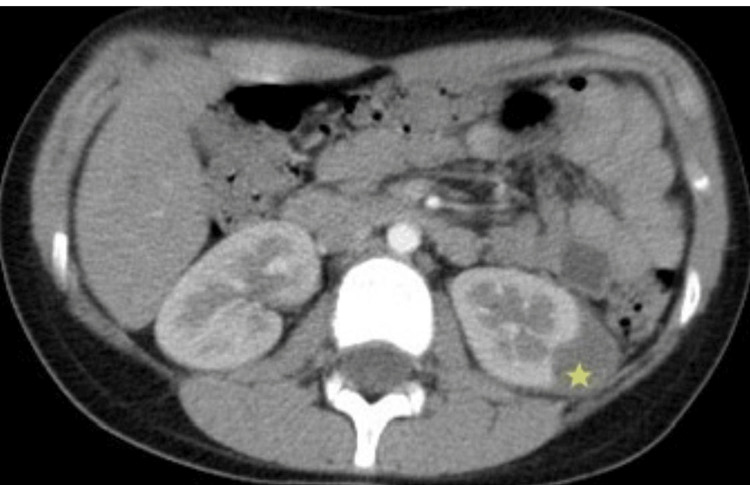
CT scan showing a reduction in size of the renal collection to 1.7 cm after two weeks of IV antibiotics (yellow star).
